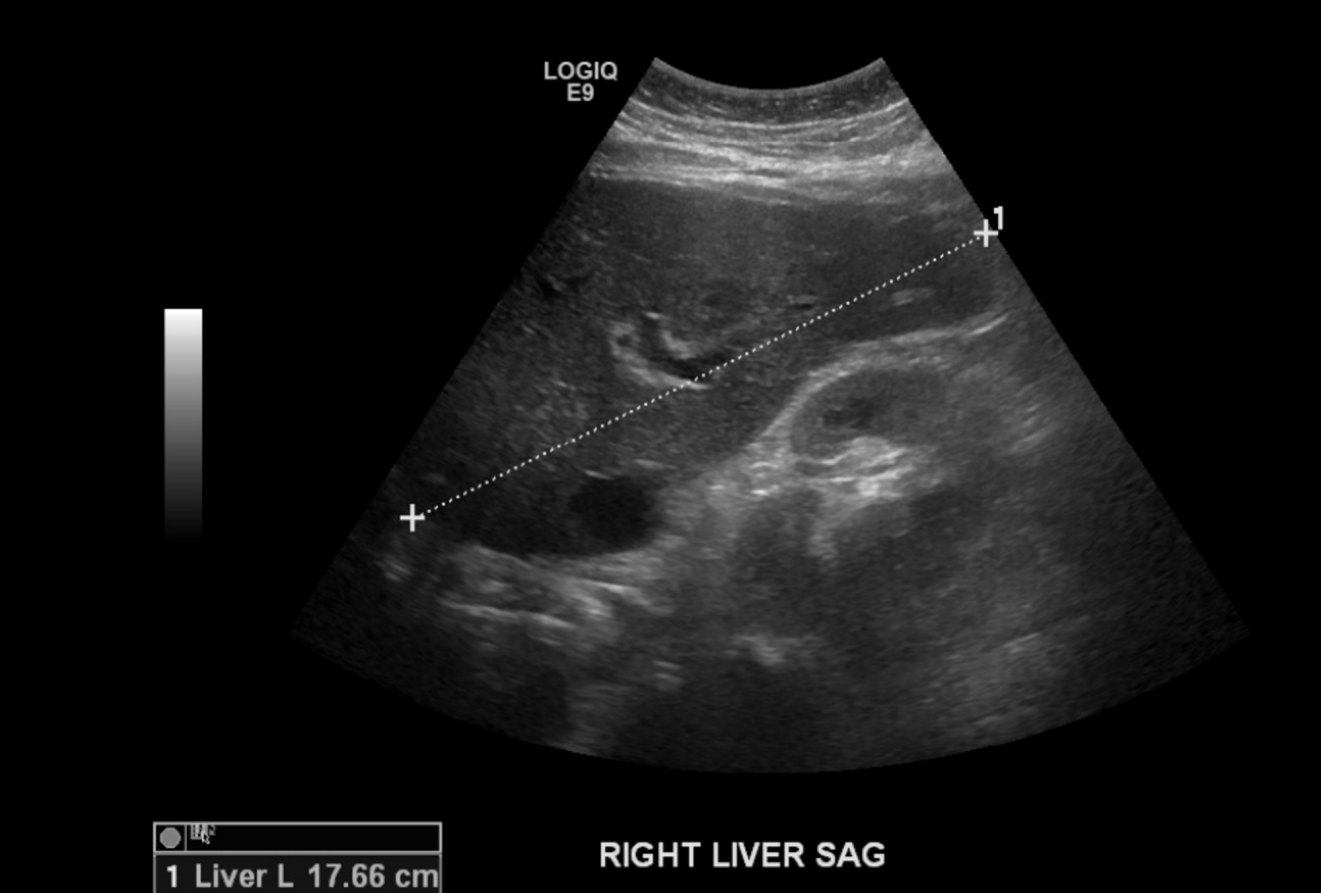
Enlarged liver on sonogram
